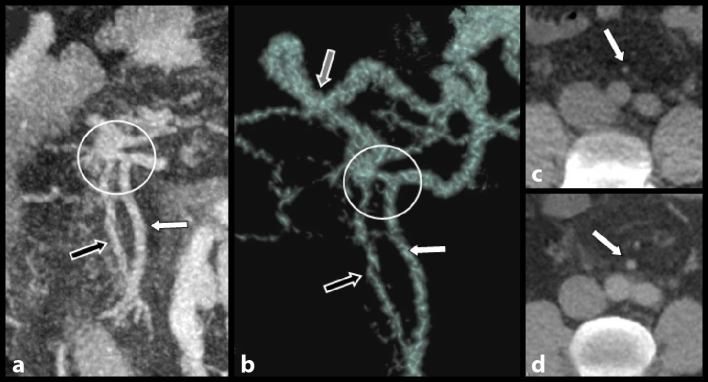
Contrast-enhanced abdominal CT images. Details of the maximum intensity projection view (a) and the volume rendering view (b) show the superior rectal artery (white arrows), the normal superior rectal vein (black arrows) and the presence of an indisputable arteriovenous communication (white circles). It is noteworthy that the caliber of the rectal vein abruptly increases just distal to this anomaly (grey arrow on b). Moreover, the caliber of the inferior mesenteric artery also appears considerably increased (white arrow on d) when compared with a view obtained 4 years ago (white arrow on c).
